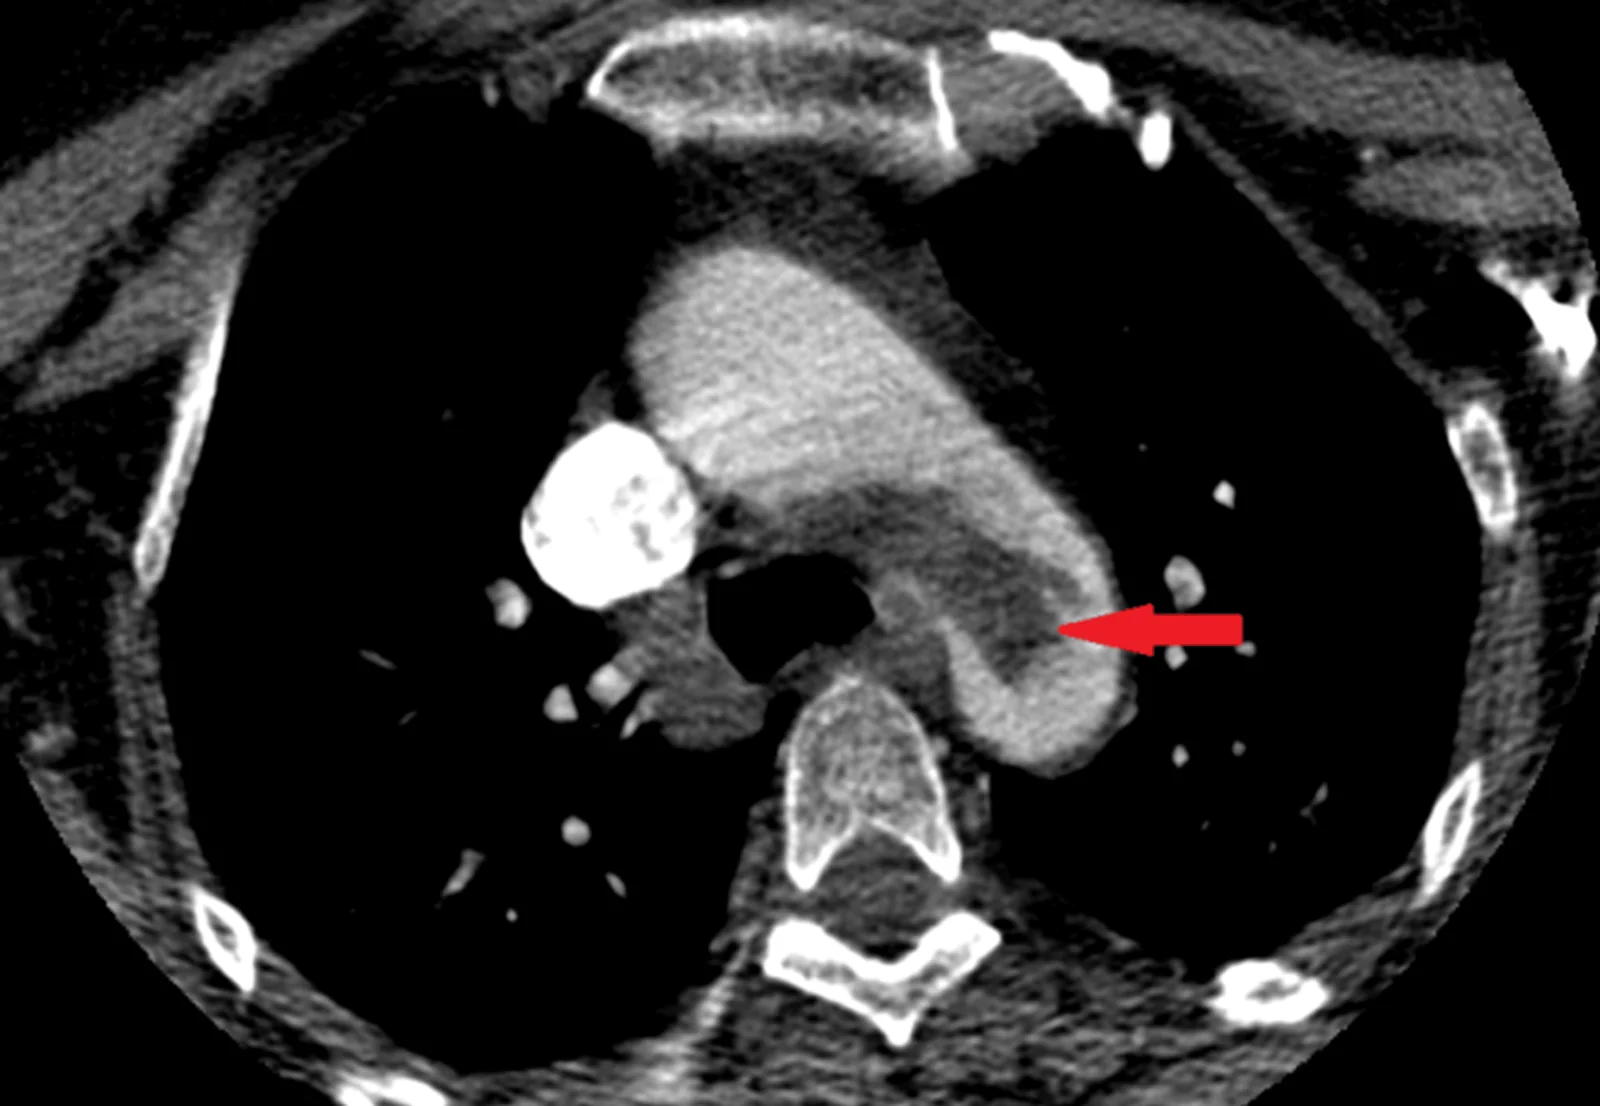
CTA showing a floating thrombus of the aortic arch in a 58-year-old woman (Case 5)
